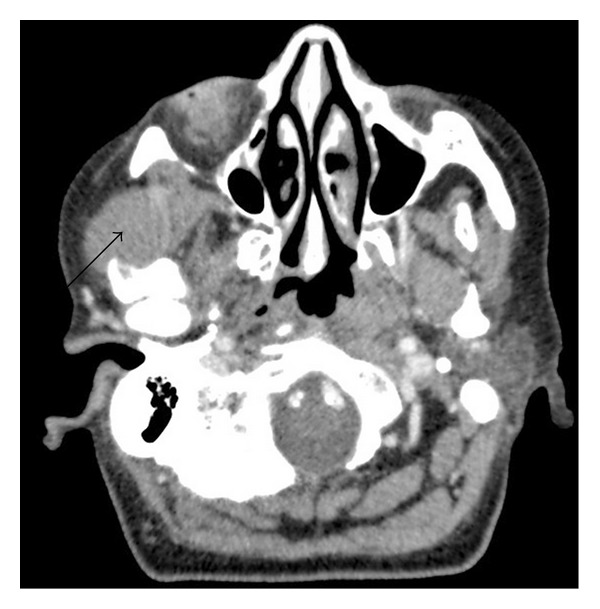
CT axial section showing tumour invading right zygomatic arch.
